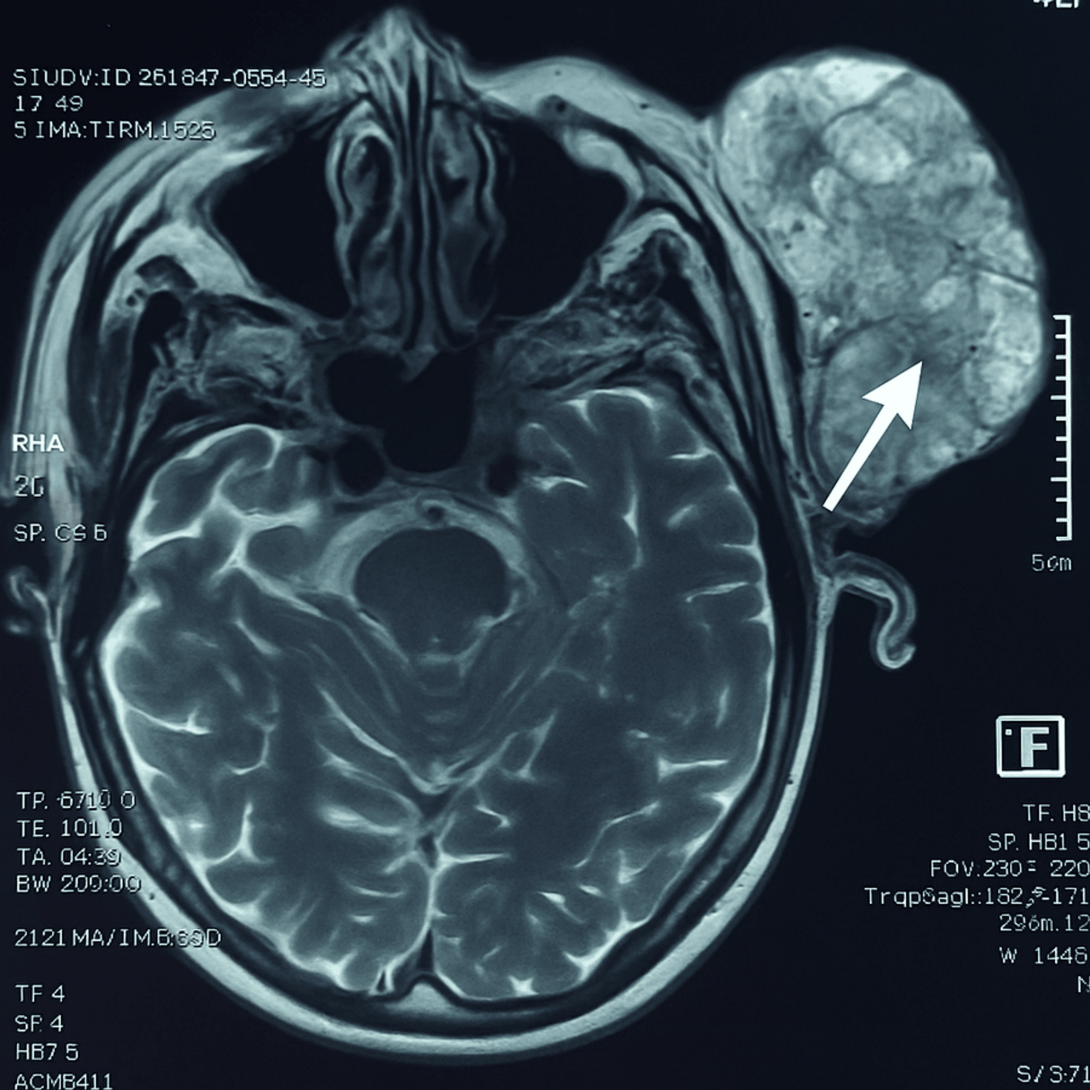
Axial T2-weighted MRI scan showing a large heterogeneous parotid mass occupying the left parotid region, consistent with locally advanced carcinoma.
